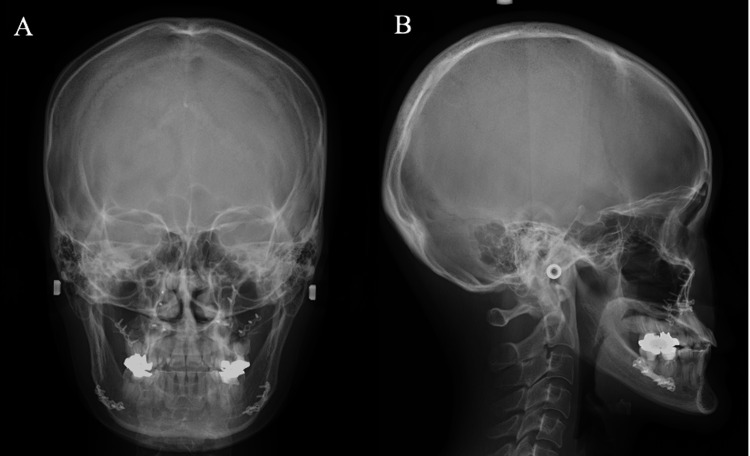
Postoperative radiographs (A) Posteroanterior cephalometric radiographs. (B) Lateral cephalometric radiographs. LeFort I osteotomy and BSSO were performed under general anesthesia. Both the upper and lower osteotomized segments were plated using titanium osteosynthesis.
